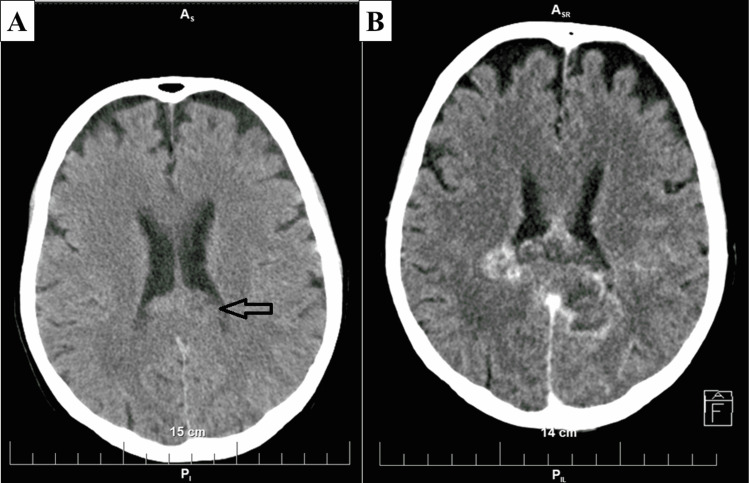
Initial CT (A) and control CT (B) (A) Isointense lesion in the splenium of the corpus callosum (arrow). (B) Bilateral tumor with irregular borders, with a significant increase in size.
